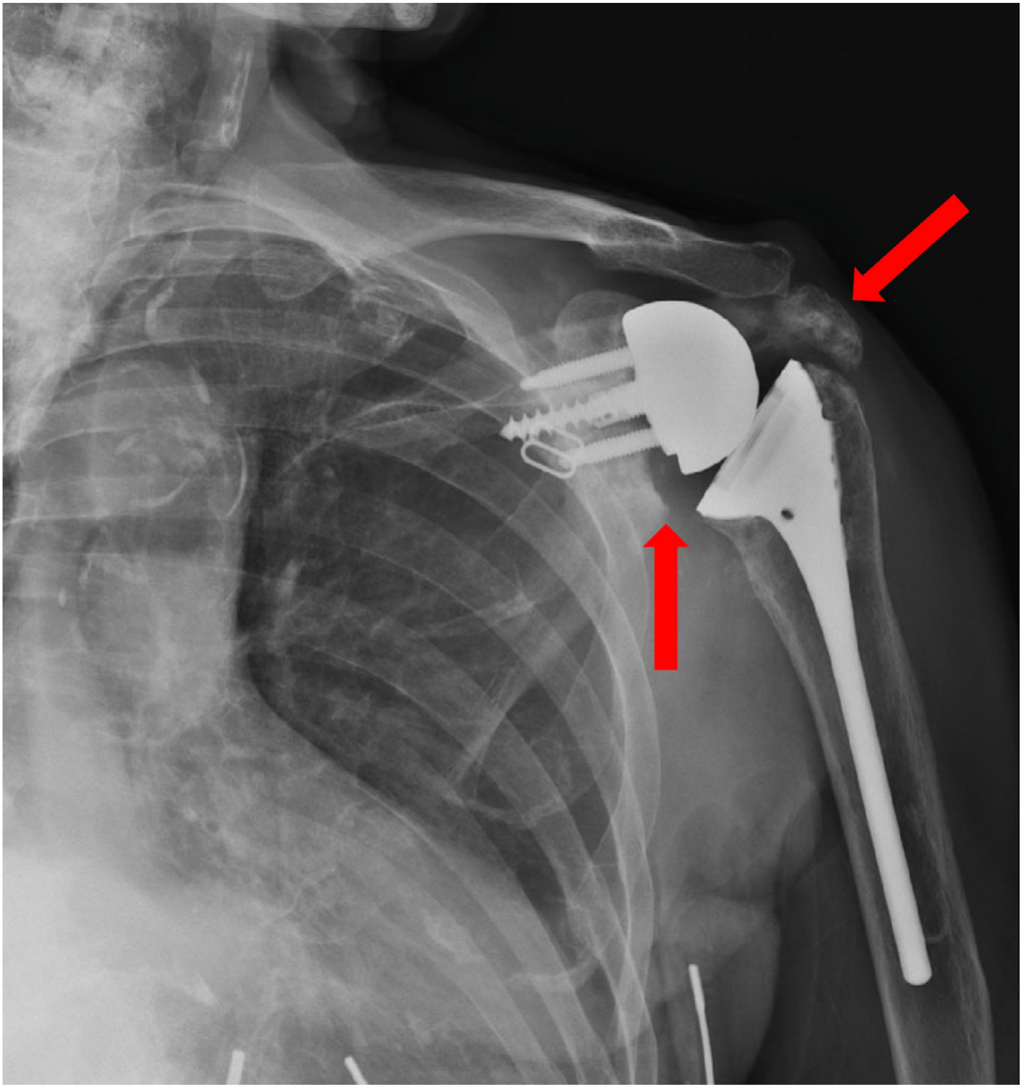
X-ray Grashey view demonstrating a change in glenoid component positioning relative to prior imaging in Fig. 10.
